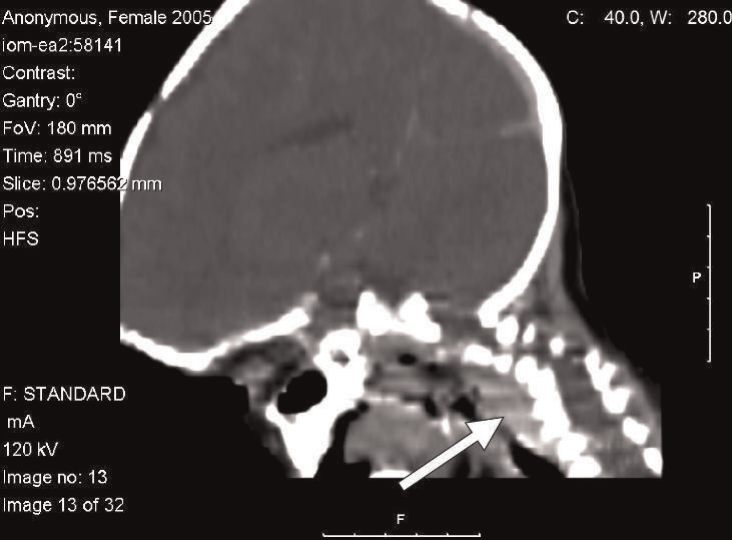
The sagittal reformation of the axial computed tomography scans (taken at time of presentation) show a retropharyngeal abscess that has tracked down from the mastoid abscess.
